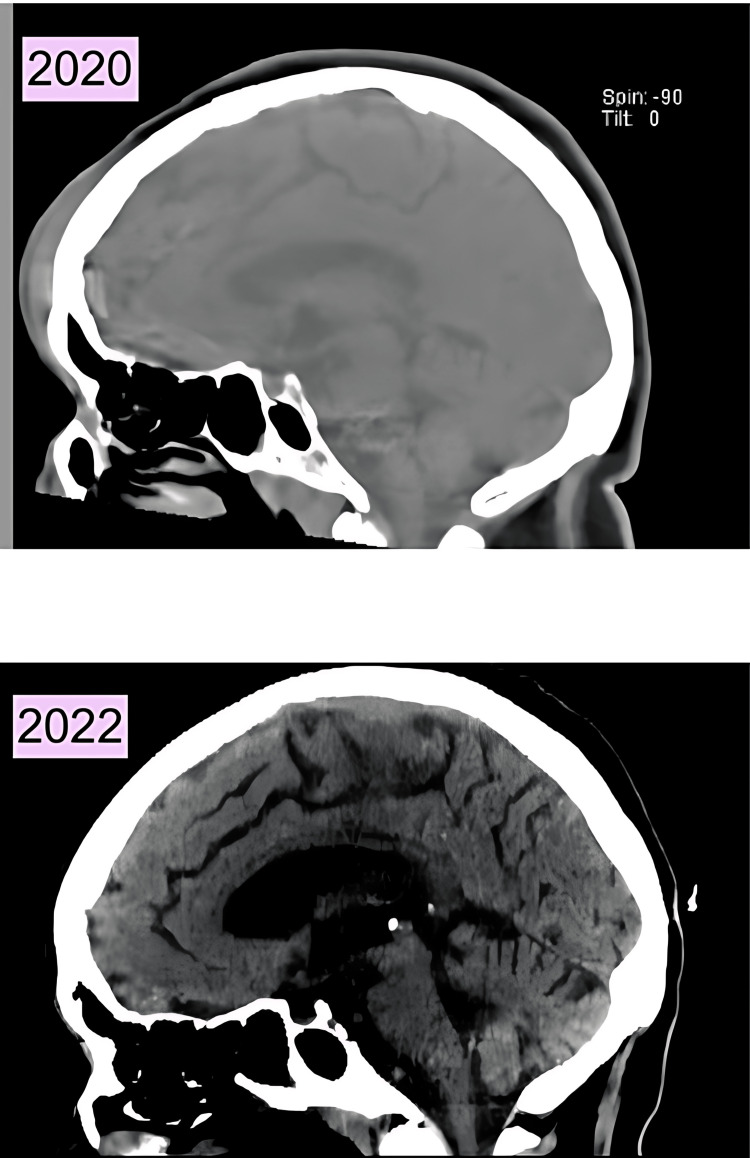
Sagittal CT without contrast scan of head done in 2022 in comparison to CT done in 2020 showing significant cerebellar atrophy.
